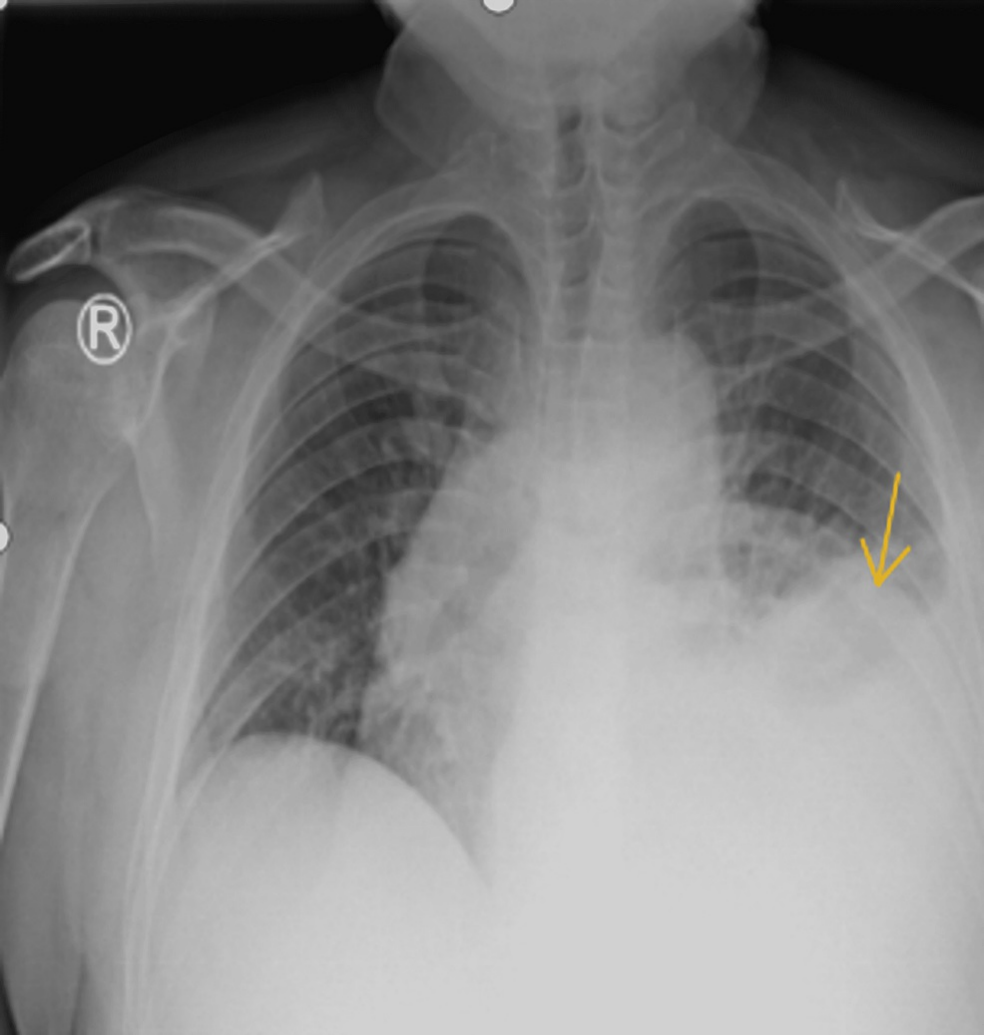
Chest X-ray showing left pleural effusion.
